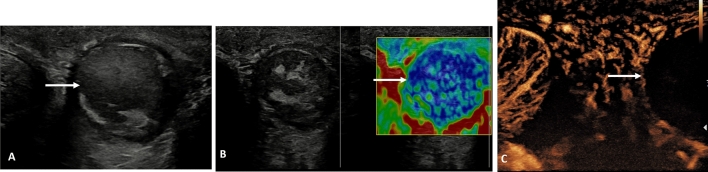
Presentation following several days of testicular pain in an 18 year old; spermatic cord torsion. A B-mode transverse view of the testis, demonstrating an enlarged heterogenous left testis (arrow). B Tissue elastography demonstrates a heterogenous color map, predominantly blue, which indicates increased stiffness (arrow). C A contrast enhanced ultrasound examination, using maximum intensity projection, confirms absence of flow in the left testis (arrow), confirming a “missed” spermatic cord torsion
